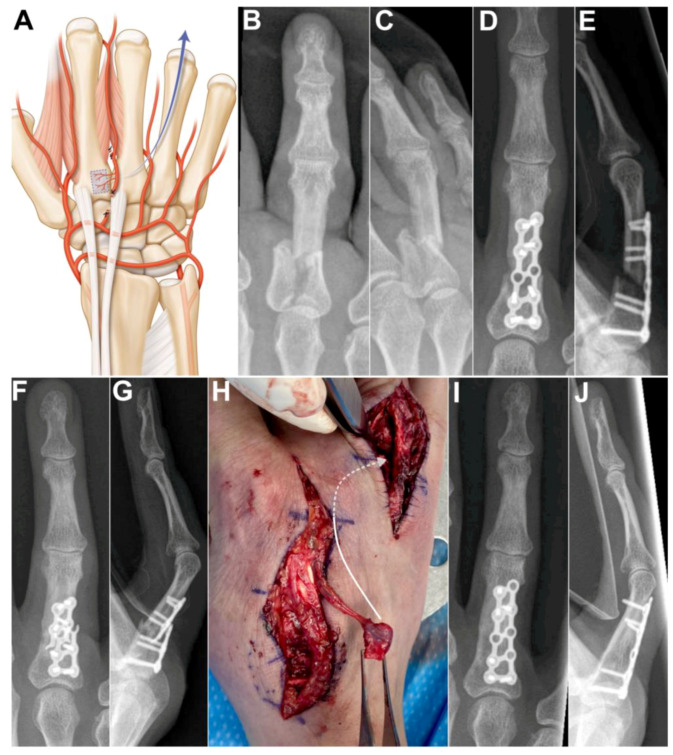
(A) Arterial anatomy, the distally based flap requires ligating the 2DMA proximally to the base of the second metacarpal as well as the palmar communicating branch; Case 4. (B,C) preoperative X-rays showing unstable and displaced intra-articular fracture of the first phalanx of the 4th ray. (D,E) postoperative X-rays, osteosynthesis with a dorsal plate and screws. (F,G) 7 months after osteosynthesis, X-rays showing unstable nonunion of the proximal phalanx after plate breakage; (H) Intraoperative photograph showing vascularized bone graft, dissection between extensor tendons and skin was made to allow passage of the vascularized 2nd metacarpal-base bone graft to the 4th ray (arrow). (I,J) postoperative X-rays demonstrating union of the phalanx.
